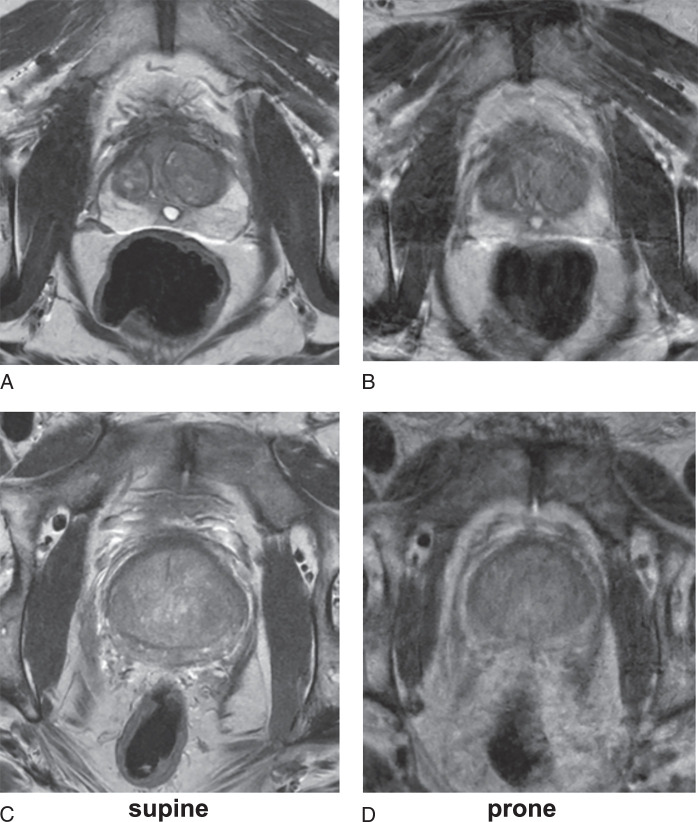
Prostate MR images from 2 different patients demonstrating inferior image quality of prone T2-weighted imaging due to motion artefact (B, D) compared with supine T2WI (A, C). PI-QUAL scores for T2 axial components: (A) 3/3, (B) 1/3 with a point scored for adequate signal-to-noise ratio, (C) 3/3, (D) 0/3.
